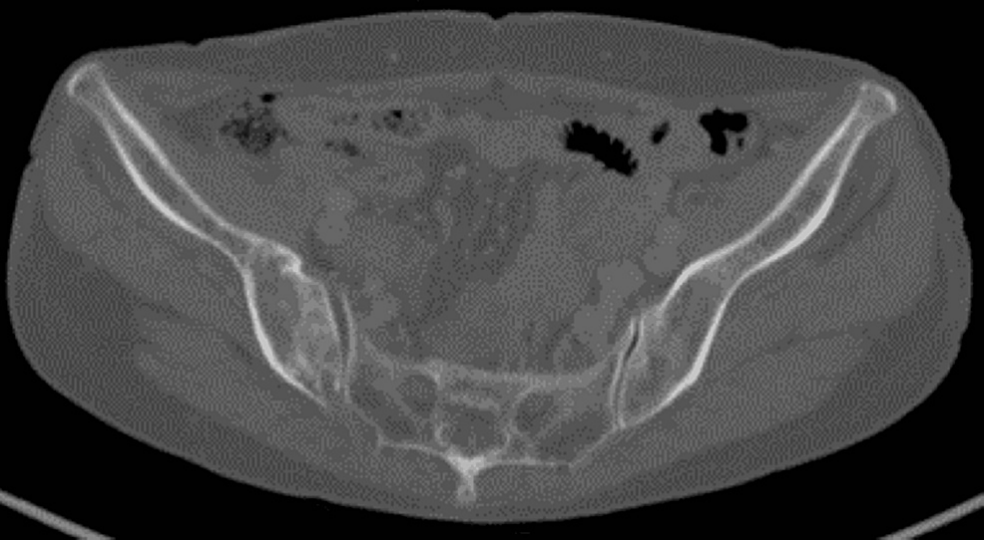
CT scan taken at six months after discharge. Osteosclerosis and fusion of the right iliac bone near the sacroiliac joint are visible.
